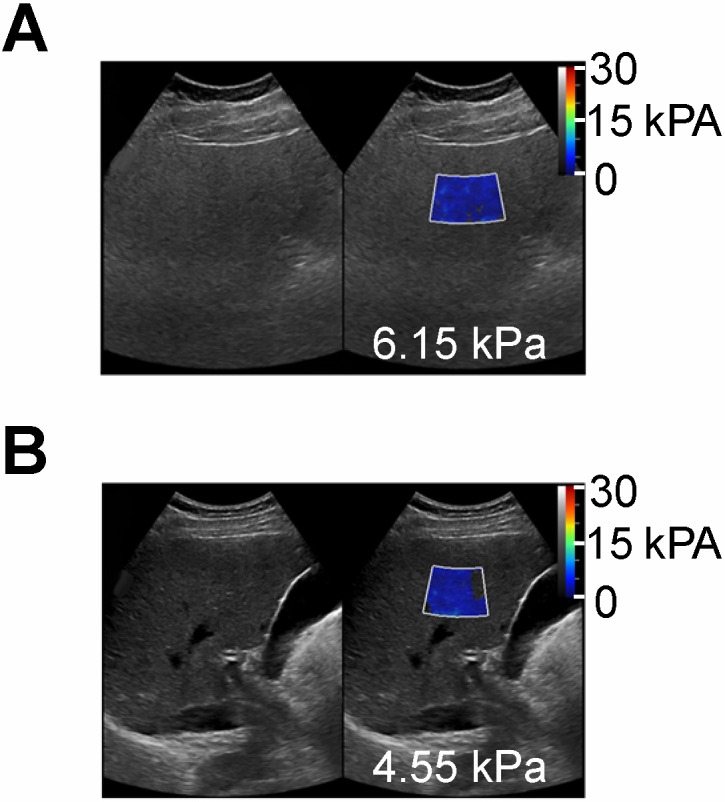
Shear wave elastography quantitative ultrasound method with calculated SWE measurements shown as color-coded scale superimposed on grayscale clinical images. (A) 55 year old female patient with NAFLD and MRI calculated fat fraction of 43 % with SWE measurement of 6.15 kPa and (B) a 60 year old male without history of NAFLD and MRI calculated fat fraction of 1.4 % with SWE measurement of 4.55 kPa. These SWE measurements show no significant differences (6.15 vs 4.55 kPa) despite marked variability in MR calculated fat fractions (43 % vs 1.4 %).”
